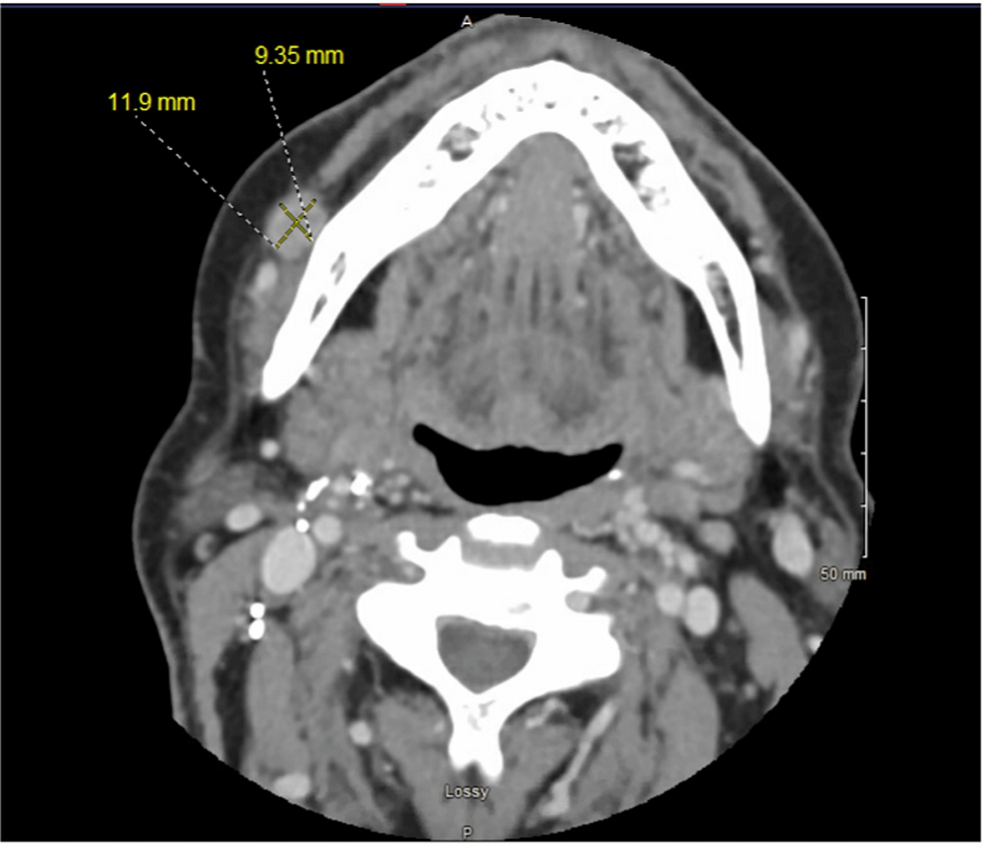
CT with contrast of the neck showing a heterogeneous enhancing 1.2 cm nodule adjacent to the right mandible. CT: computed tomography
